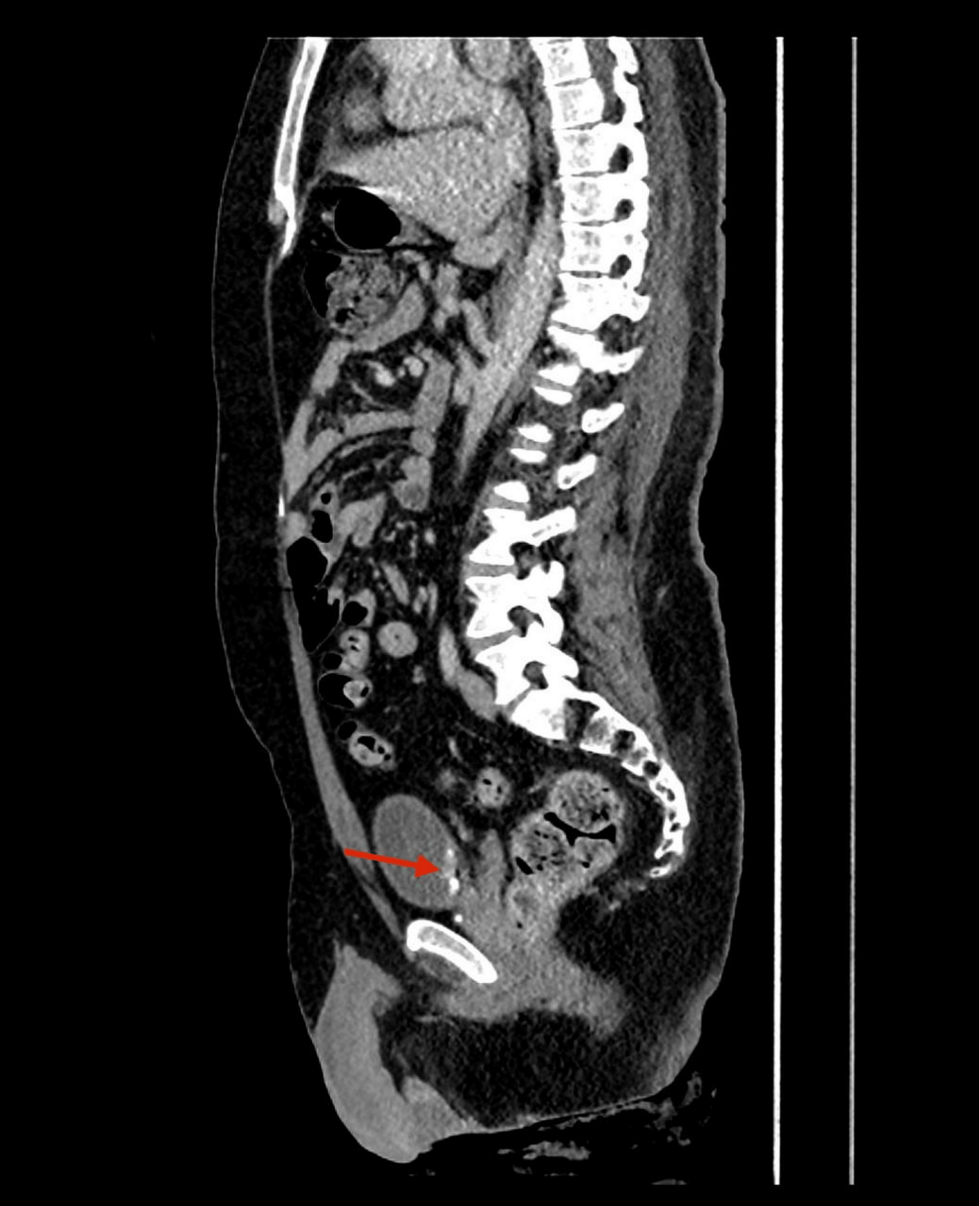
CT urography (sagittal view) showing a heterogeneous enhancing mass (arrow) arising from the left bladder wall. The tumor projects into the bladder lumen as a filling defect with irregular contours. The sagittal plane highlights the depth of bladder wall involvement. Abnormal finding: irregular intravesical mass with wall distortion. Normal findings: no evidence of spread outside the bladder, with surrounding pelvic structures preserved.
